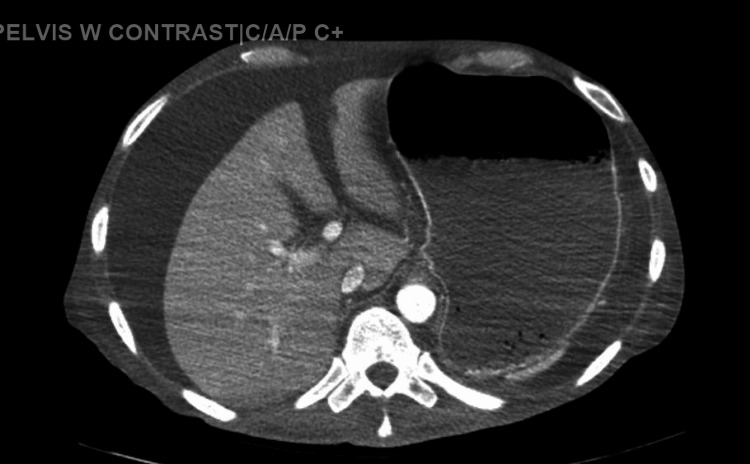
CT image showing ascites fluid around the liver with an enlarged stomach
